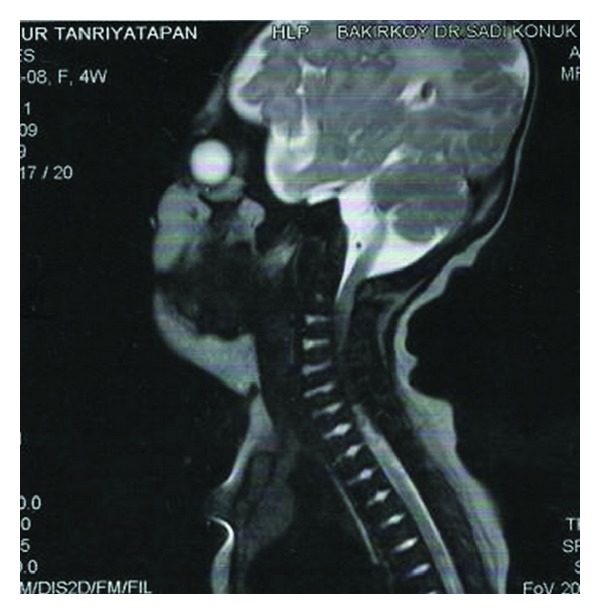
On the cervical spinal MRI, spinal stenosis at C4-5, C5-6, and C6-7 levels.
